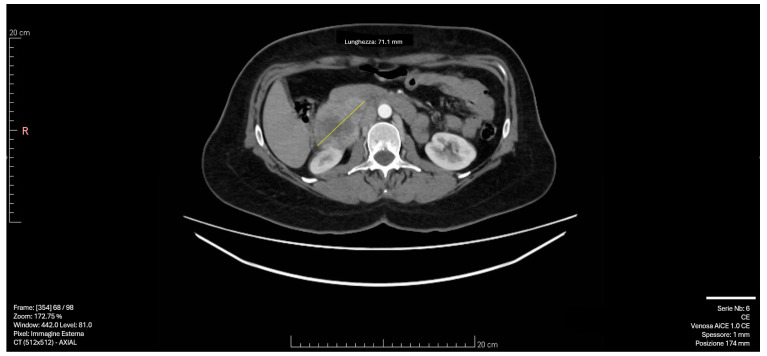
CT scan image: The right adrenal mass demonstrated adjacency to but no infiltration of the inferior vena cava, with venous drainage observed in the right ovarian vein. The yellow arrow indicates the 55 × 50 mm solid formation with a necrotic core and irregular peripheral enhancement in the right adrenal lodge.
